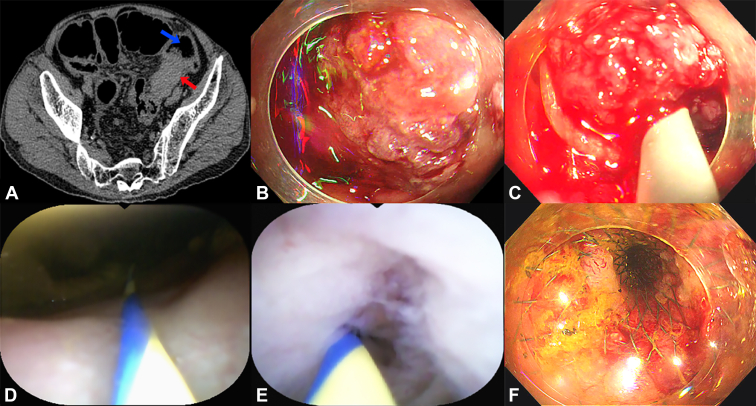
The imaging examination and radiation-free peroral cholangioscopy-guided self-expandable metal stent placement for sigmoid colon cancer with intestinal obstruction. A, Computed tomography examination suggested a sigmoid colon tumor (*red arrow*) causing secondary intestinal obstruction (*blue arrow*, dilated colon proximal to the tumor). B, Colonoscopy showed a very friable sigmoid tumor mass causing severe colonic stricture. C, The peroral cholangioscope (white catheter) identified the narrowing in the stricture and was advanced through this area. D, The guidewire crossed the stenosis to reach the proximal dilated bowel lumen. E, Observed tumor stenosis during peroral choledochoscope withdrawal. F, Colonic stent placed across sigmoid stricture.
